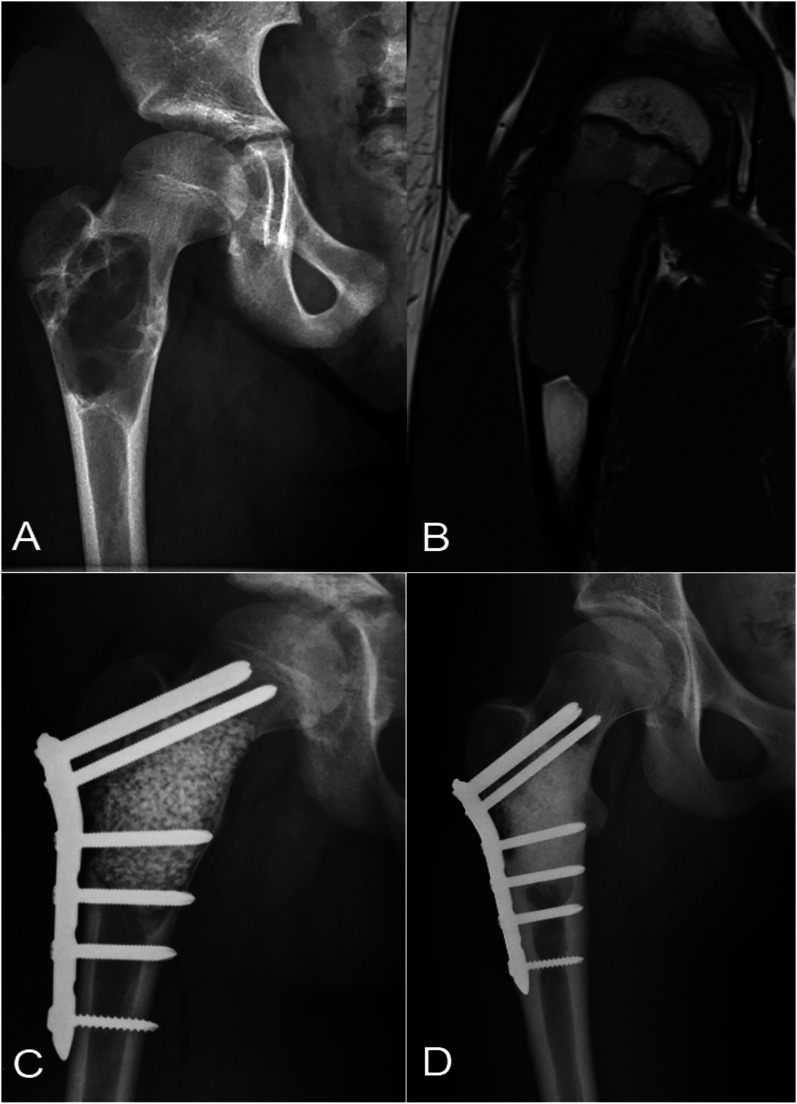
Figs. 3-A through 3-D An aneurysmal bone cyst in the proximal femur of an 11-year-old girl. Fig. 3-A Preoperative radiograph. Fig. 3-B Preoperative T1-weighted MRI. Fig. 3-C Radiograph after intralesional curettage, filling of the defect using bioactive glass, and locking-plate osteosynthesis. Fig. 3-D Radiograph showing a recurrence at the 2-year follow-up.
